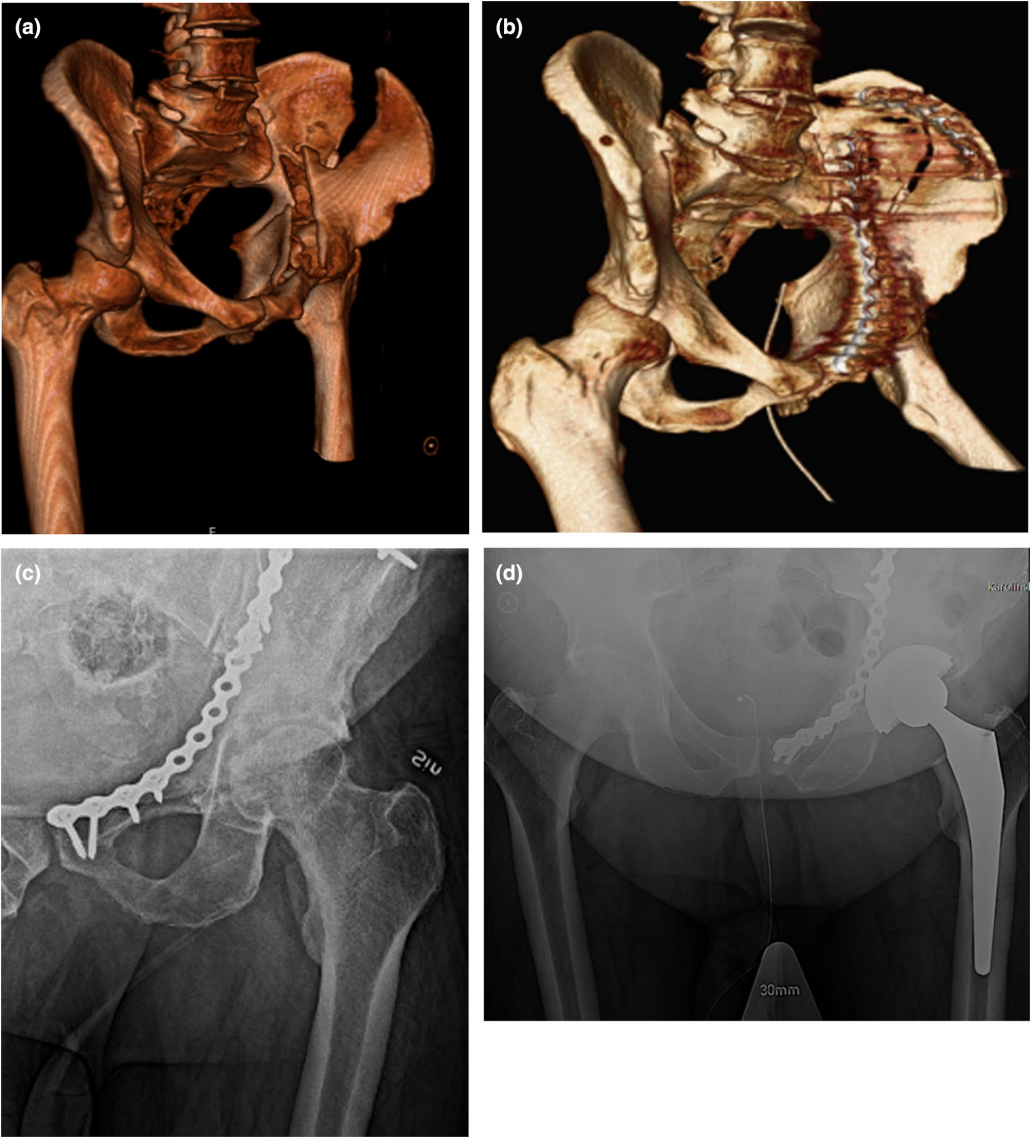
a CT scan of a 62-year-old male who sustained an anterior column + posterior hemi transverse acetabular fracture after a low-energy fall. b Postoperative CT scan after treatment with plate fixation. c Radiograph 3 years later displaying signs of arthrosis and avascular necrosis of the femoral head. d Postoperative radiographs after reoperation with an uncemented THA
